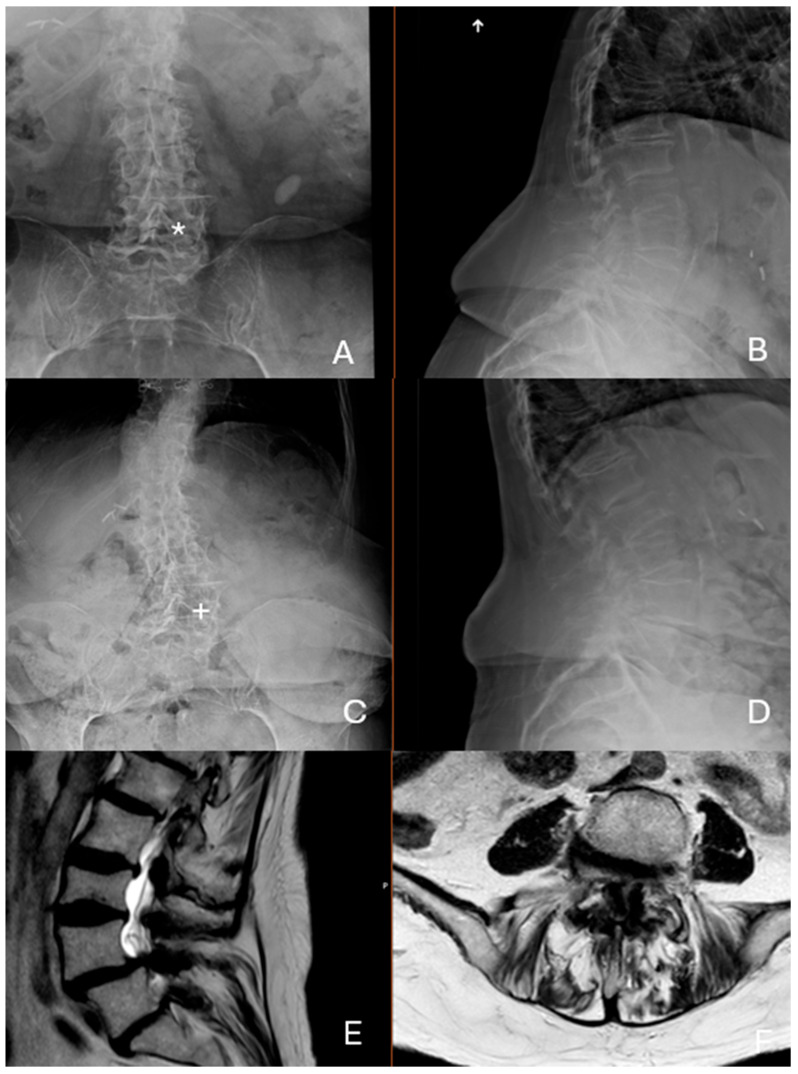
An 85-year-old female with L4–5 spondylolisthesis, severe central canal stenosis, and lost ability to ambulate due to pain severity. (A): Preoperative AP radiograph demonstrating lumbar scoliosis and severe osteoporosis with a small left sided interlaminar window (*). (B): Preoperative lateral radiograph showing an L4–5 spondylolisthesis. (C): 6-month postoperative radiograph demonstrating the left side bony resection (+) needed for the unilateral laminectomy with bilateral decompression. (D): Lateral radiograph demonstrates there is no slip progression at 6 months. (E): Preoperative T2-weighted sagittal MRI showing the L4–5 spondylolisthesis and severe central canal stenosis. (F): Preoperative T2-weighted axial MRI demonstrates severe central canal stenosis.
